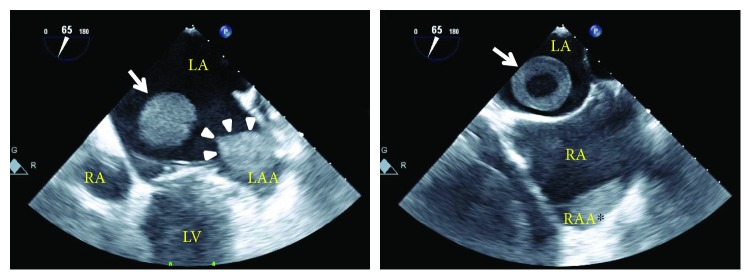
Two-dimensional transesophageal echocardiographic images of the free-floating ball-shaped mass (arrows), left (arrow heads), and right (^∗^) atrial appendage thrombi. LA: left atrium; LAA: left atrial appendage; LV: left ventricle; RA: right atrium; RAA: right atrial appendage.
